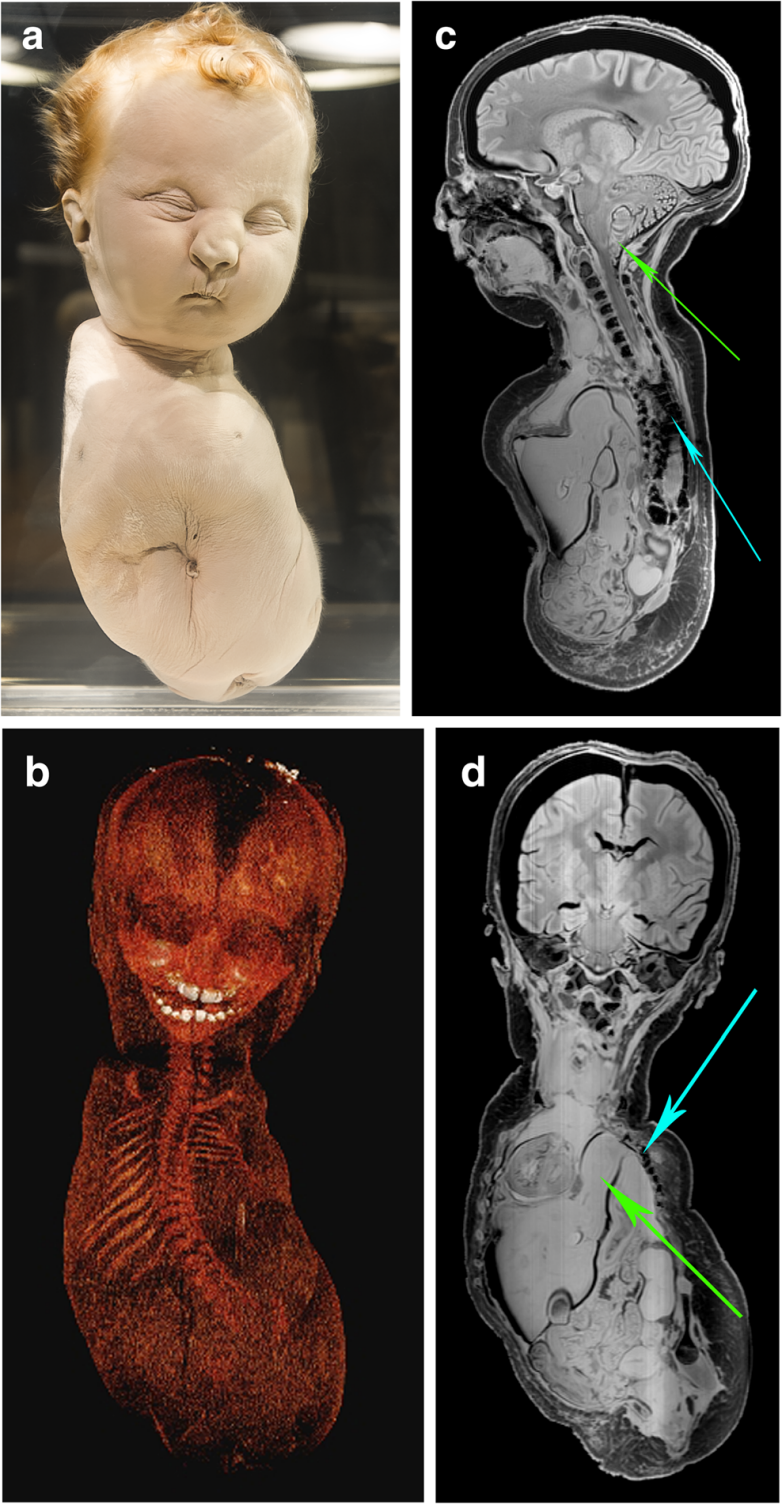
a Photograph of the full-term fetus of case 4. b Three-dimensional reconstructed skeleton based on the CT data. Although there was severely diminished bone calcification, CT images visualised small pelvic bones, partial absence of the sacral bone and a thoracolumbar convex shaped curvature. The scapulae and clavicles were normally developed with absence of the humeral, ulnar and radial bones including the hands. In addition, the femora, tibial and fibular bones including the feet were absent. c Sagittal T1-weighted MR image showed an Arnold-Chiari malformation (*green arrow*) and distorted vertebral column (*turquoise arrow*). d Coronal T1-weighted MR image showed a severe diaphragmatic hernia (*green arrow*) which deviated the heart completely to the right lateral thoracic wall. The left pleural cavity was almost entirely occupied by the liver, stomach, spleen and left adrenal gland, the left lung was merely noticeable. Moreover, a distortion of the left ribs was seen (*turquoise arrow*)
